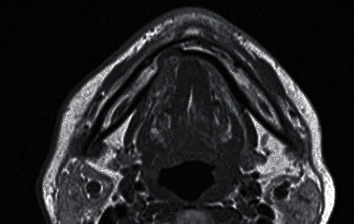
Magnetic resonance imaging, horizontal cross section, T1-weighted imaging. A rounded cavity is observed in the lingual side of the mandible from the right anterior to the premolar region. The margin of the cavity in the lingual side of the mandible exhibits a cortical bone structure, and the cavity's margin is smooth. A layer of myeloid tissue is observed in the cavity between the lingual cortical bone and the buccal cortical bone. The tissue in the cavity in the lingual side of the mandible has similar signal intensity to the sublingual glands and is contiguous with the sublingual gland tissue.
